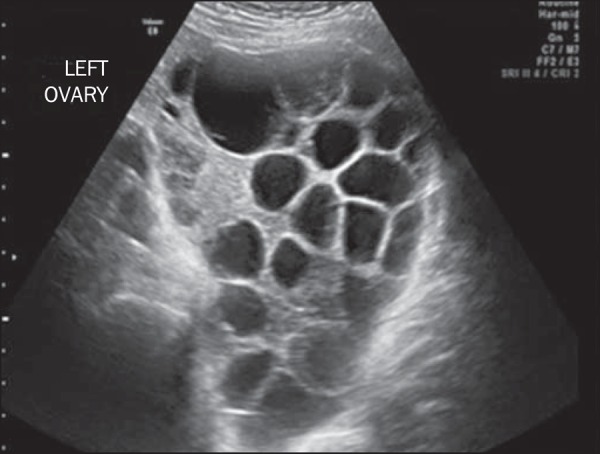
Pelvic ultrasound showing a massive theca lutein cyst in a patient
